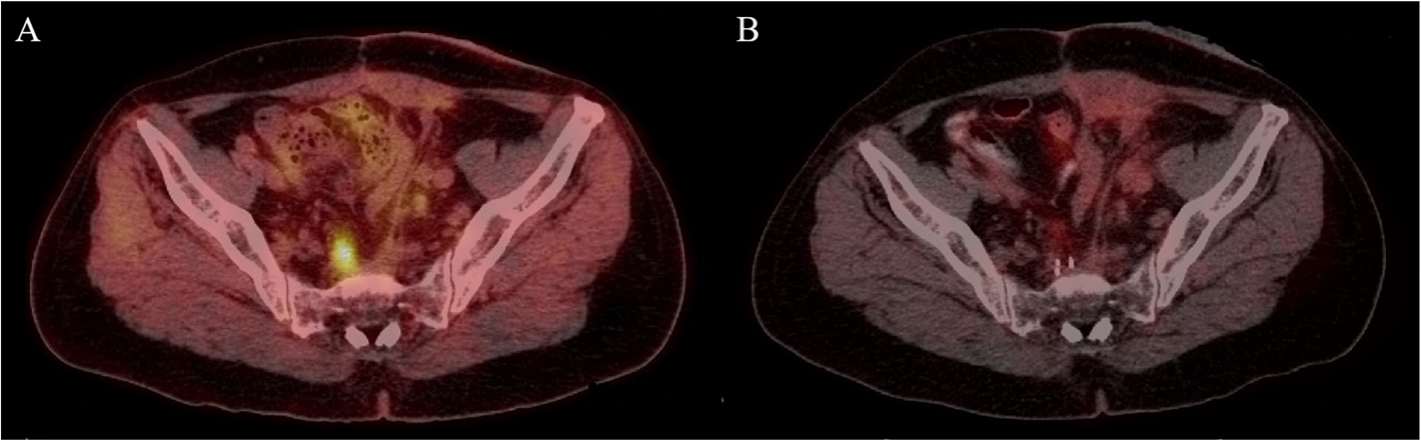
Stage III, T3N2M0, adenocarcinoma, presacral recurrence, previous Dixon’s operation, EBRT: DT 50.4 Gy before. PET-CT images (A) before seeds implantation, (B) 3 months after seeds implantation. EBRT: external beam radiotherapy; PET-CT: positron emission tomography-computed tomography
